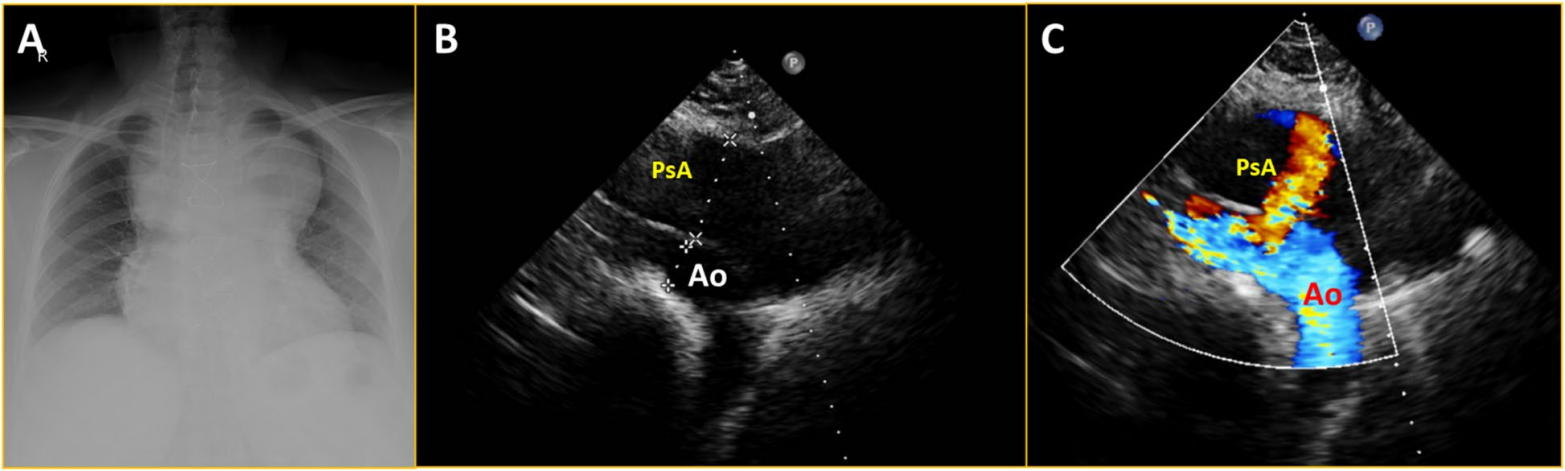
Chest X-ray and 2D echocardiogram. A Chest X-ray showing huge mediastinal mass. B, C Modified parasternal view showing huge pseudoaneurysm anterior to aorta with discrete neck of the pseudoaneurysm with turbulent flow within. PsA = pseudoaneurysm; Ao = aorta
